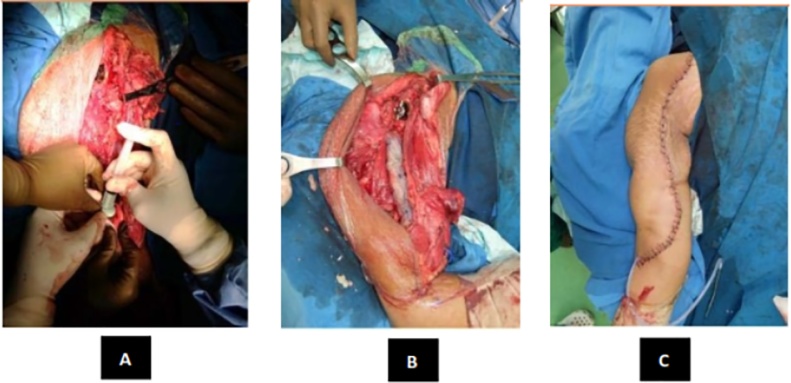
(A) Bone Cement Application (B) Mess Application (C) Wound Closure. Post-operative radiograph was taken and showed (Fig. 10).
